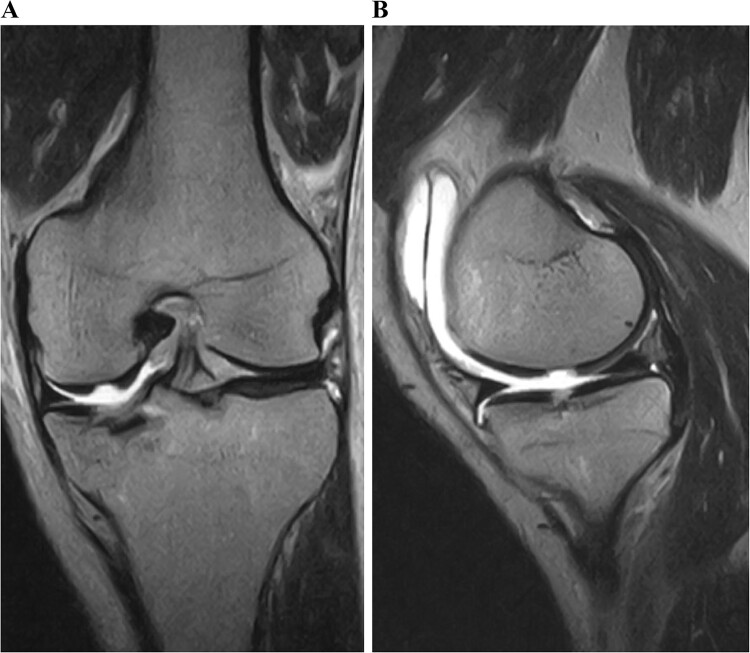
Preoperative T2-weighted magnetic resonance images of the left knee. A cartilage injury of the medial femoral condyle appeared as a kissing lesion opposite the tibial plateau fracture. The coronal image revealed a 20-mm-wide cartilage injury (A). The sagittal image showed an 18-mm-long cartilage injury (B).
